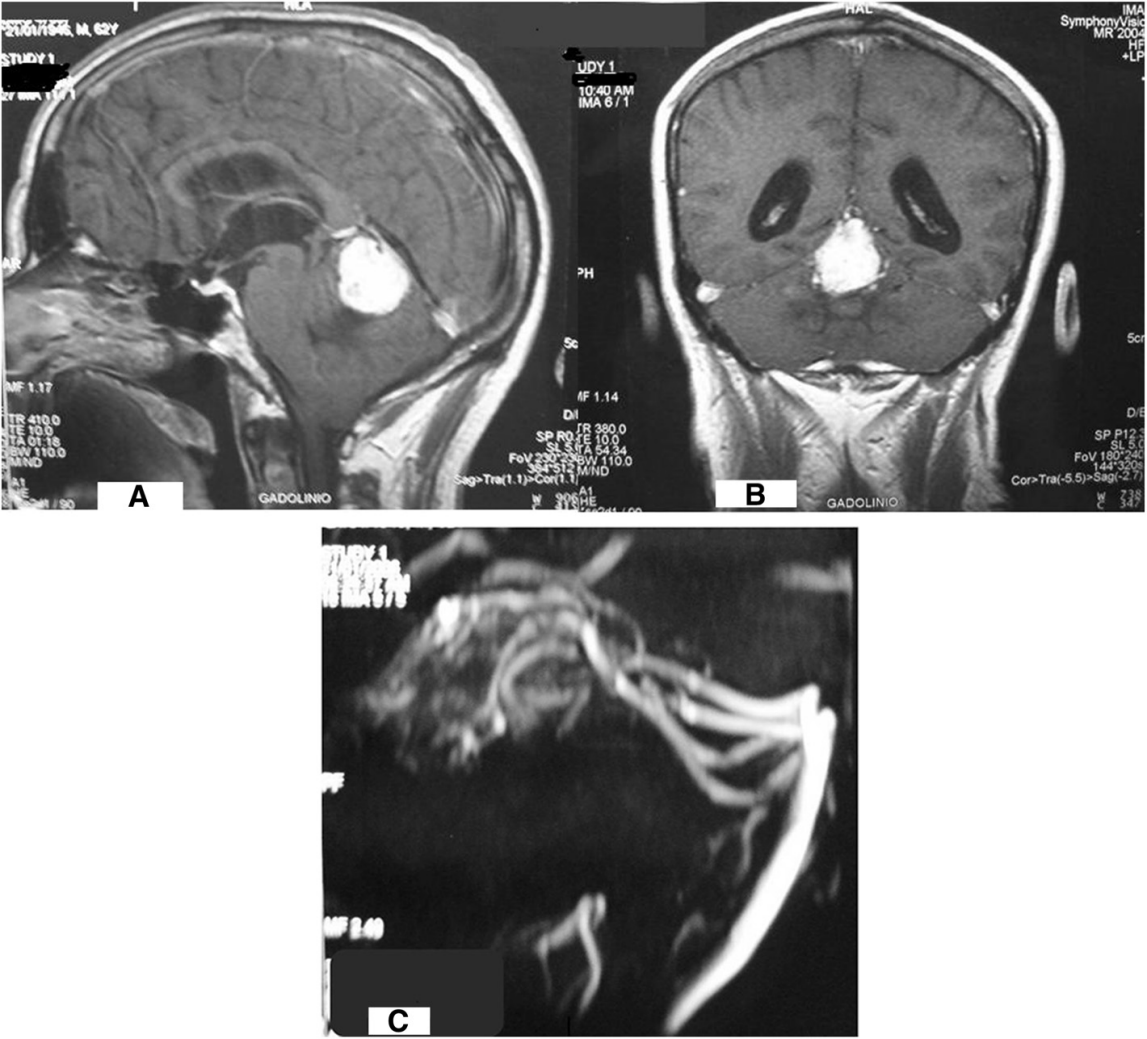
a and b Gadolinium-enhanced T1-weighted magnetic resonance imaging demonstrated a homogeneously enhancing mass in the posterior incisural space. Coronal and sagittal views. c Magnetic resonance angiography showing dilated veins arising from the mass, consistent with a highly vascularized lesion
